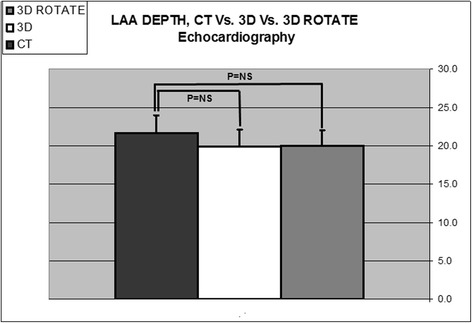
Histogram comparing LAA depth using different imaging methods (*n* = 41). Comparison between computed tomography (CT) (21.7 ± 6.9 mm), direct Real-Time 3-Dimensional Transesophageal Echocardiography (3D) (19.8 ± 2.2 mm), and Rotational 3DTEE (3D Rotate) (20.0 ± 2.1 mm), *(p* = NS for all)
